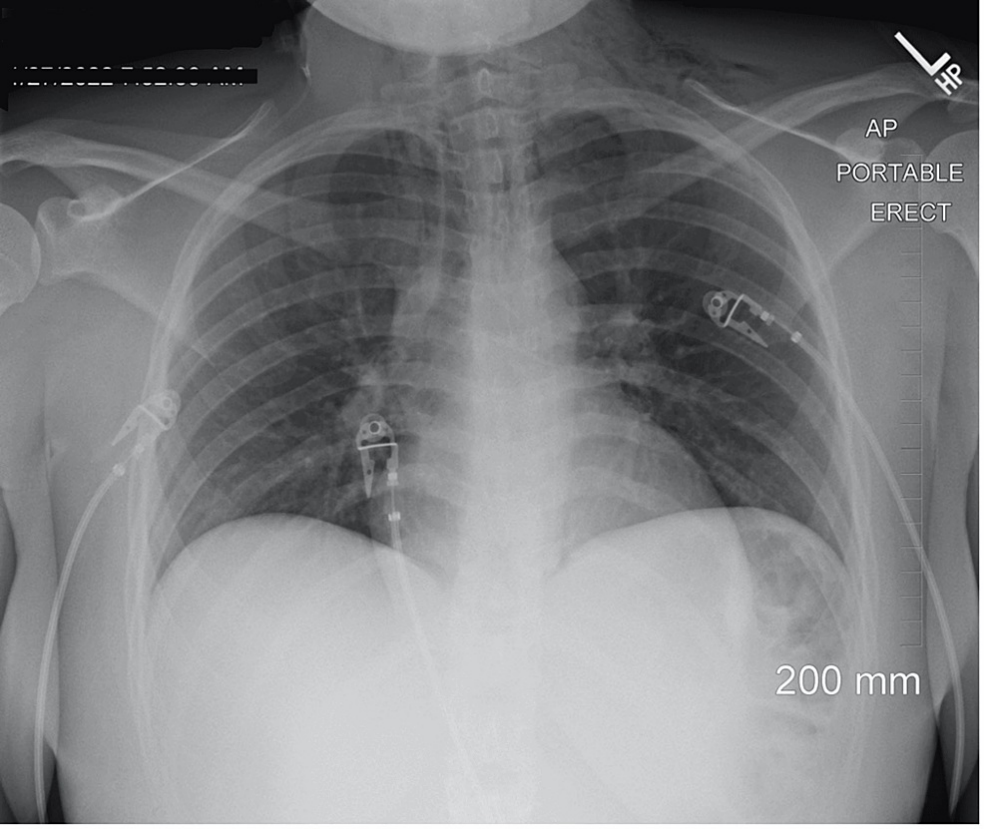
Repeat chest X-ray showing the resolution of free air in the mediastinum and cervicothoracic junction
